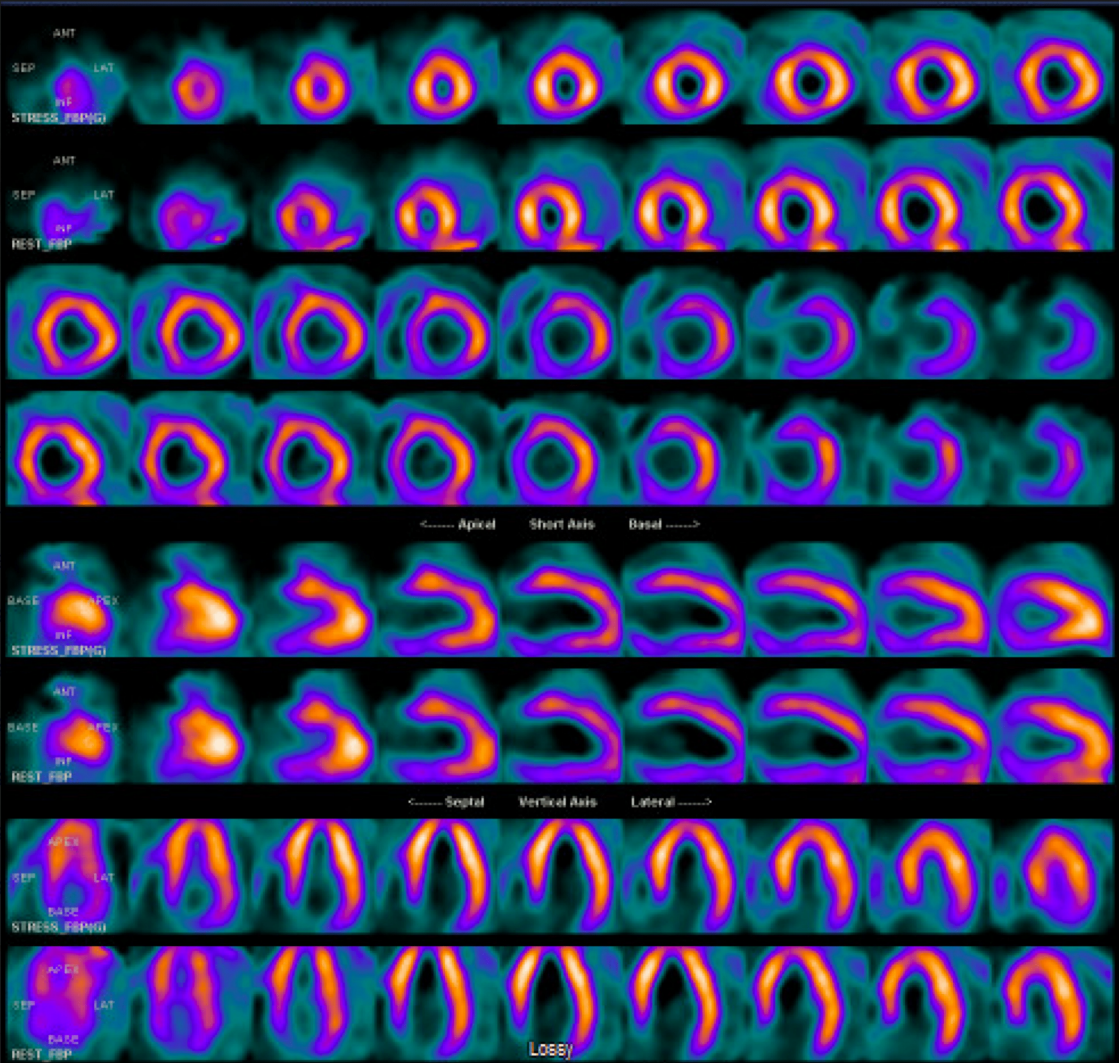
Nuclear medicine myocardial perfusion SPECT SPECT: single-photon emission computed tomography
